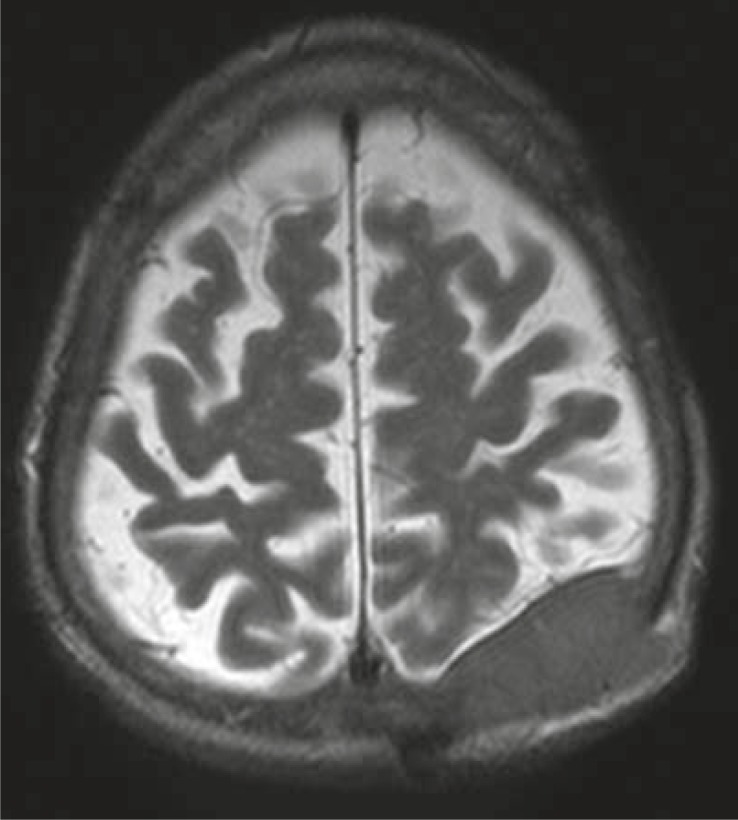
Brain MRI with a recurrent subcutaneous metastasis from hepatocellular carcinoma.
